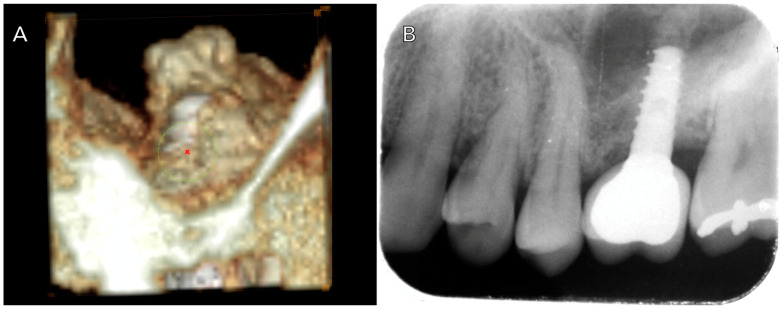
(A) Intrasinusal image showing bone formation at the expense of the sinus at 5 months postoperatively; (B) periapical radiograph at 4 years follow-up.
